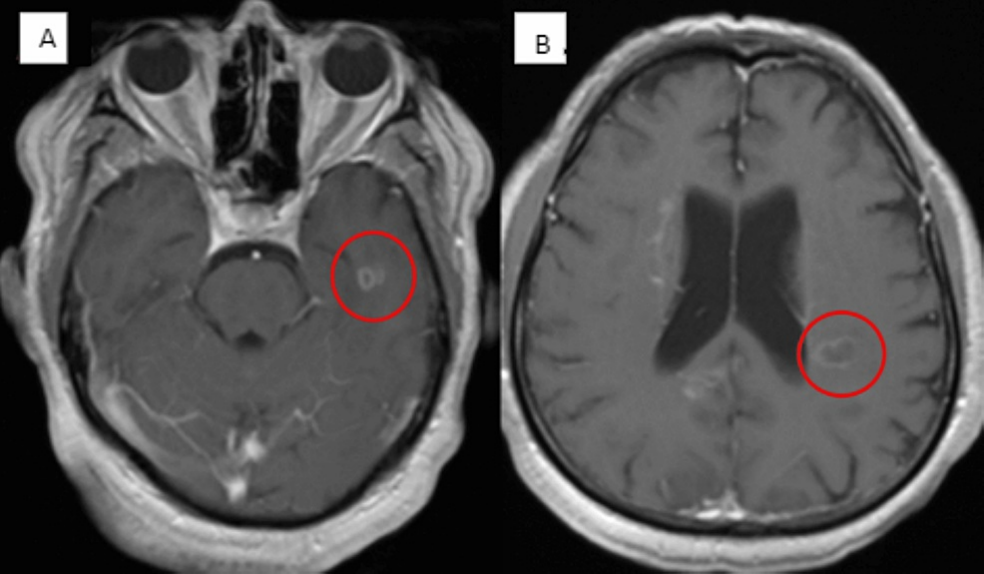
Brain MRI showing ring-enhancing lesions on T1 in the left temporal lobe (A) and left periventricular area (B) in a patient with a positive MOG antibody. MOG: myelin oligodendrocyte glycoprotein.
